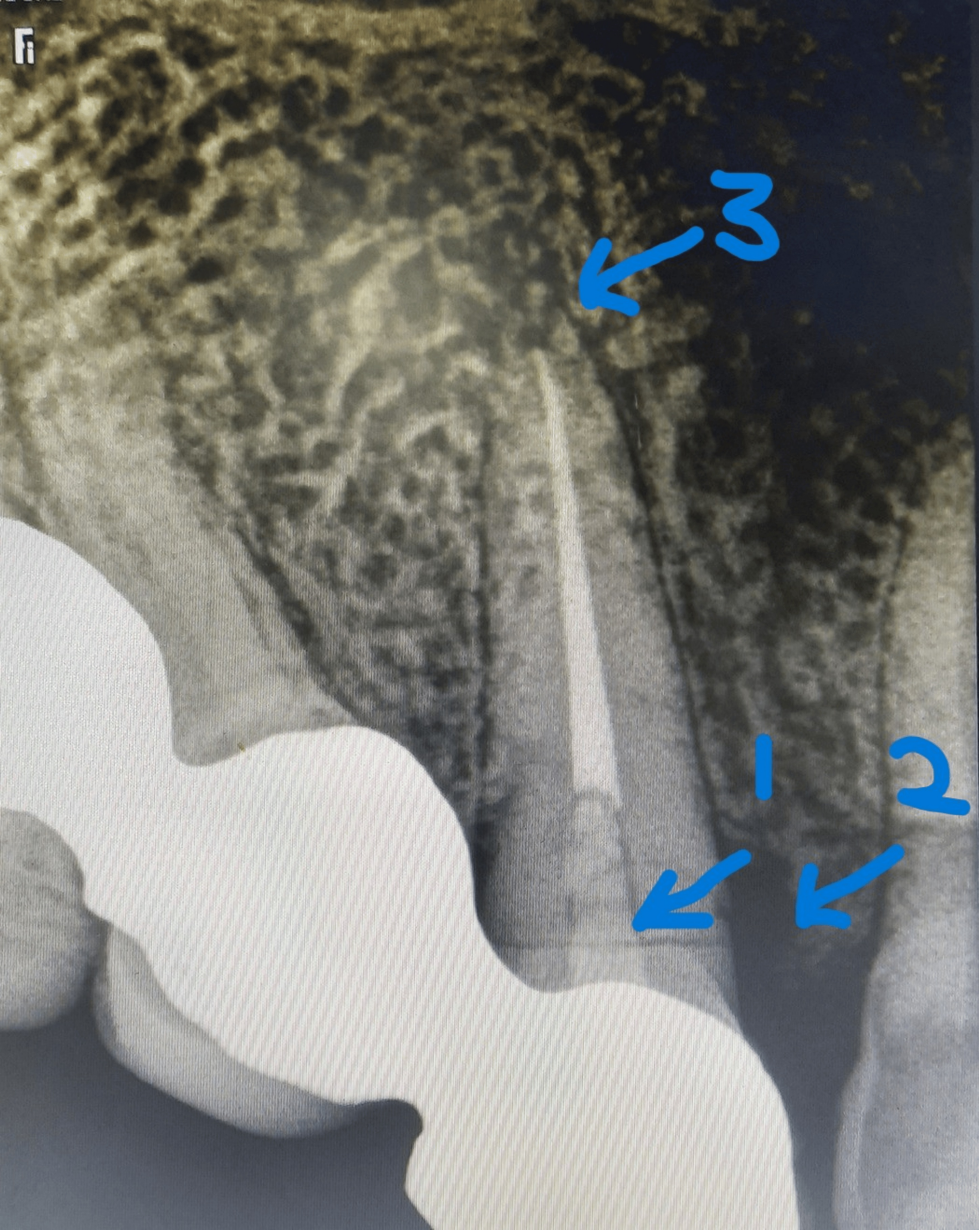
Follow-up IOPAR at six months showing evidence of ongoing healing at the fracture site (indicated by arrow 1) and crestal bone loss at the same site (indicated by arrow 2), with no evidence of periapical radiolucency (indicated by arrow 3). IOPAR: intraoral periapical radiograph
